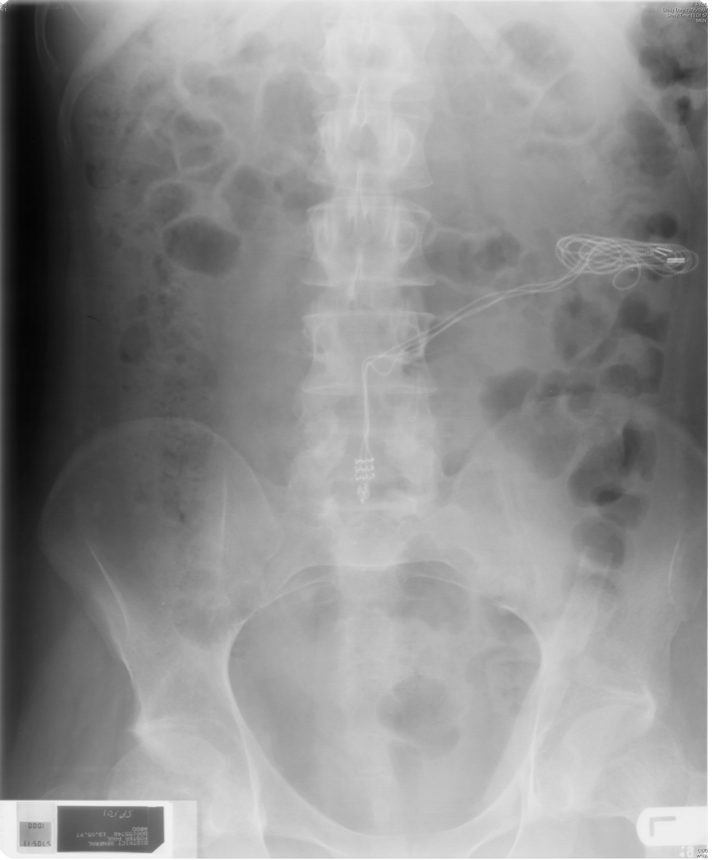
X-ray of abdomen, taken on 13 May 1997, showed the cables tunnelled in left flank. Receiver block had been removed. Please compare with Figure 1.
